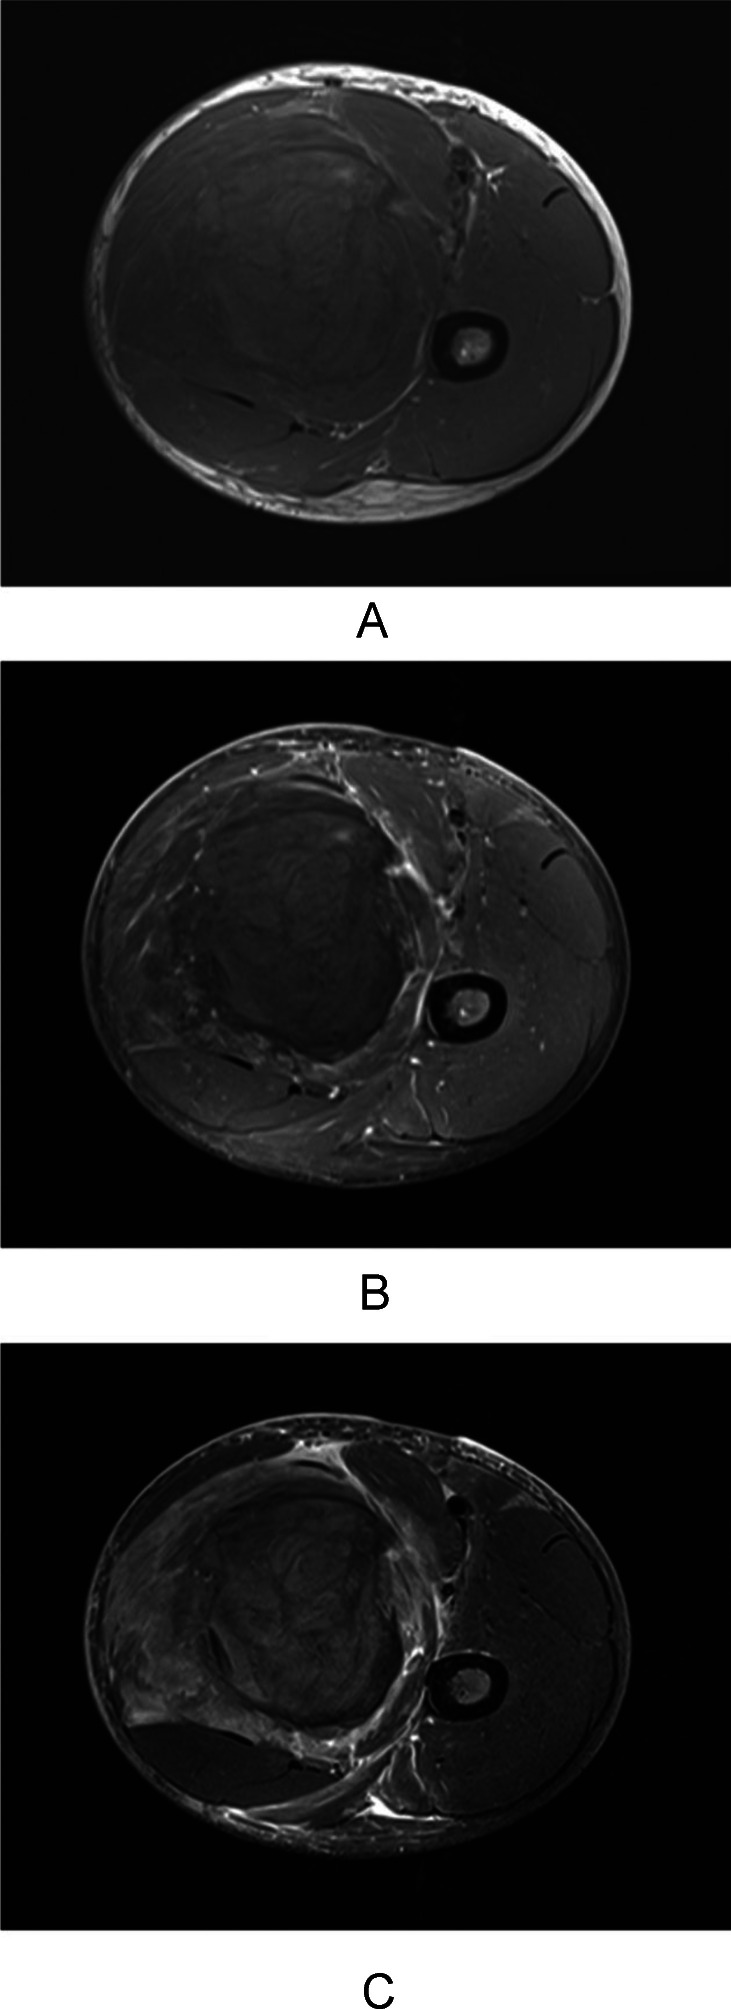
Axial T1-weighted (A), axial postcontrast fat-suppressed T1-weighted (B), and axial T2-weighted STIR (C) MRI images demonstrate a heterogeneous intramuscular hematoma within the left adductor compartment with internal blood products and extensive surrounding edema. No suspicious internal enhancement is identified.
